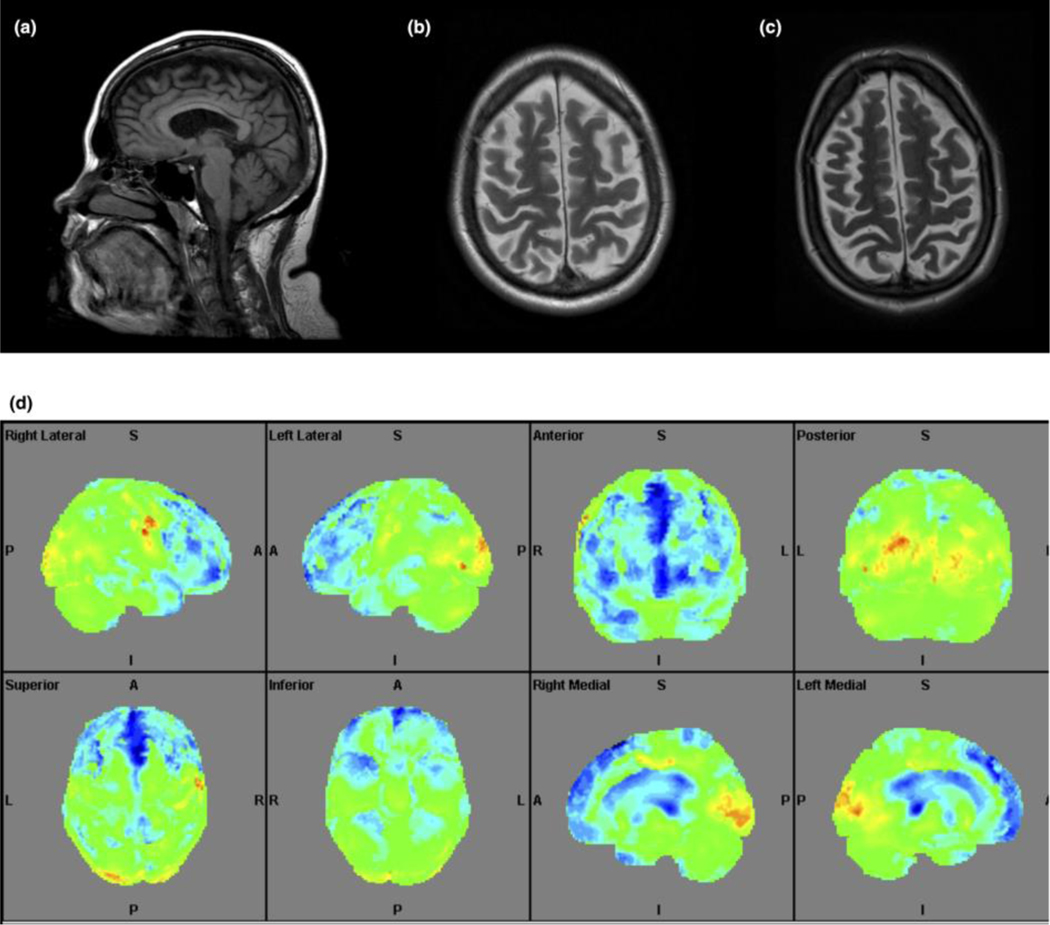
Brain MRI in Patient III:1 at 43 and 47 years of age (a) and 47 years of age (b and c). (a) Subtle atrophy of the vermis (sagittal T1-weighted imaging, 4 mm thick slices). (b) Moderate but progressive cortical atrophy (axial T2-weighted imaging, 4 mm thick slices; images not aligned similarly making the difference hard to appreciate on one slice). (d) 3D-SSP figure displaying marked hypometabolism in the PFC, part of temporal lobe and anterior cerebellum. Cingulate gyrus and thalamus are no shown.
